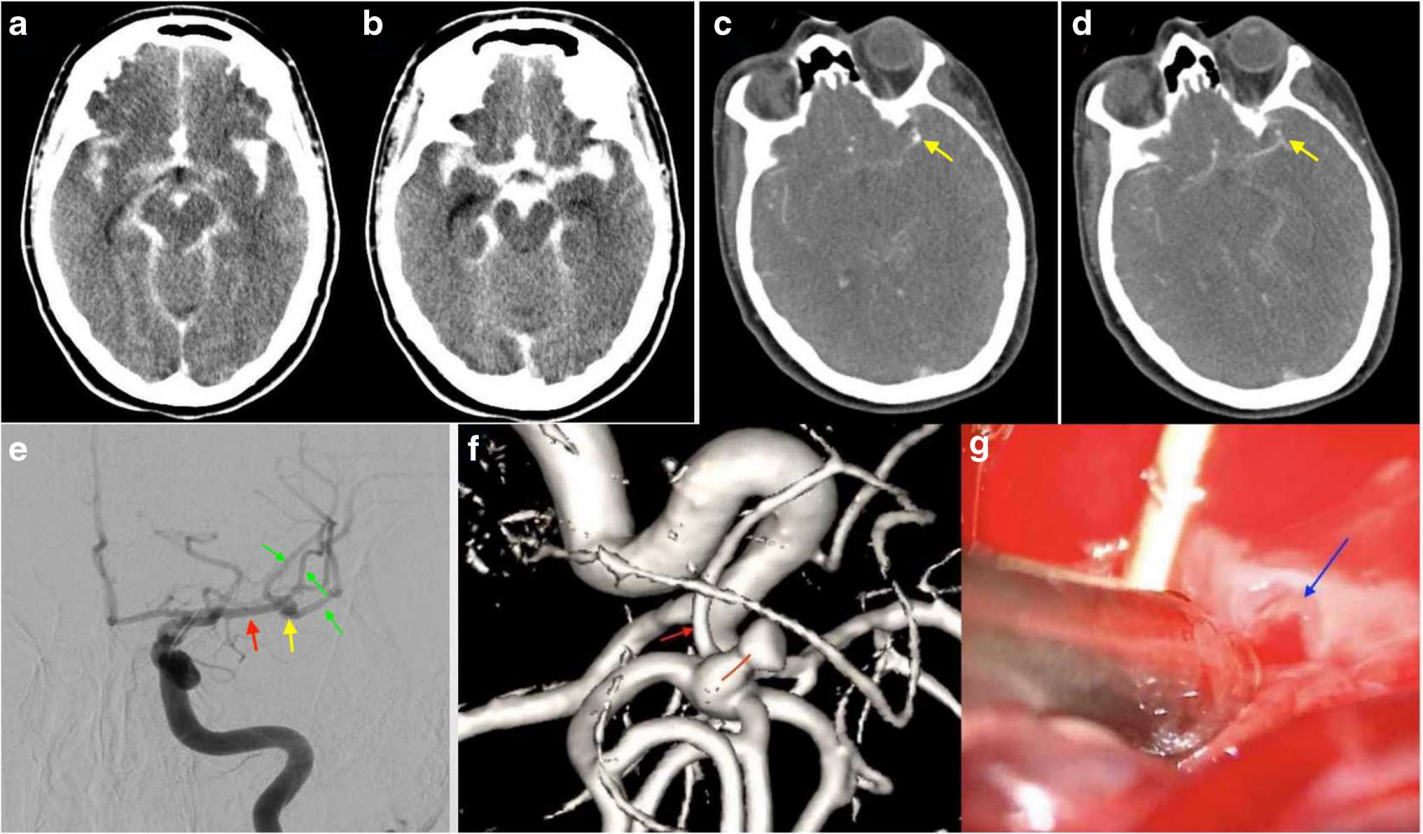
Preoperative imaging assessment of the MCA aneurysm. A and B A preoperative CT scan reveals a substantial subarachnoid hemorrhage, accompanied by the presence of a cisternal blood clot. C and D Preoperative CTA scan providing insight into the suspected position of the MCA aneurysm. E Preoperative DSA confirms the precise location of the aneurysm (indicated by the yellow arrow), with additional annotations highlighting M1 (red arrow) and M2 (green arrow). F Visualization of the partially torn neck of the aneurysm (depicted by the red line). Noteworthy is the proximity of a perforator artery originating from the M2 branch, emphasized by the red arrow. G Detailed view of the torn site of the aneurysm base, with the delicate and vulnerable area highlighted by the blue arrow
